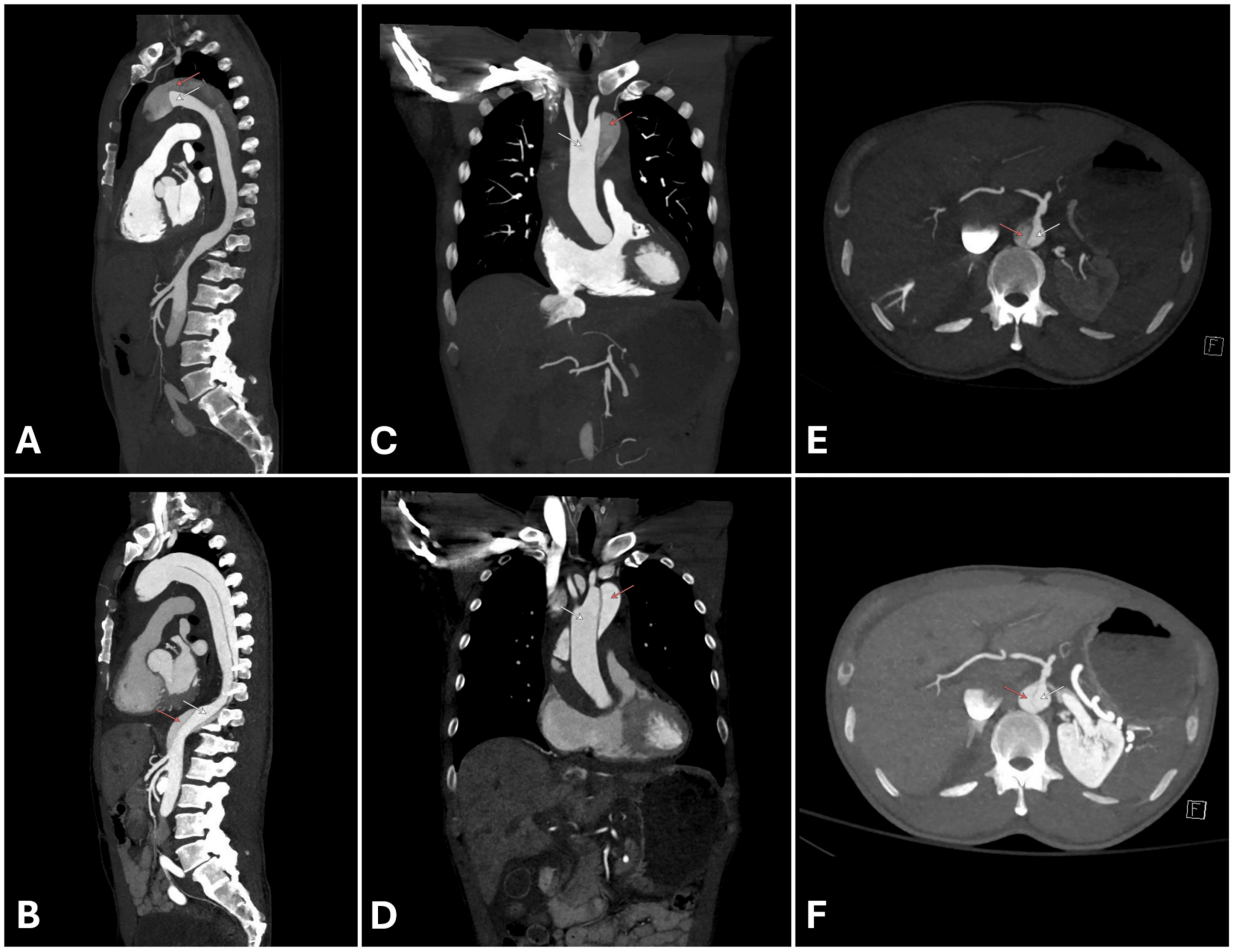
Contrast-enhanced CT angiography demonstrating Stanford type A aortic dissection Early (A) and late (B) arterial-phase sagittal multiplanar reconstructions demonstrate an intimal flap originating in the ascending aorta and extending through the aortic arch and descending thoracic aorta into the infrarenal abdominal segment. Late-phase imaging further delineates the separation of the true (white arrow) and false (red arrow) lumens. Early (C) and late (D) arterial coronal reconstructions show dissection of the ascending aorta and arch with differential luminal opacification. Reflux of contrast into the inferior vena cava is present, consistent with elevated right-sided pressures. (E) Axial imaging at the level of the celiac axis demonstrates the dissection flap adjacent to the celiac origin, with preserved opacification of the vessel. (F) Corresponding late arterial axial imaging further highlights the distinction between the true (white arrow) and false (red arrow) lumens
